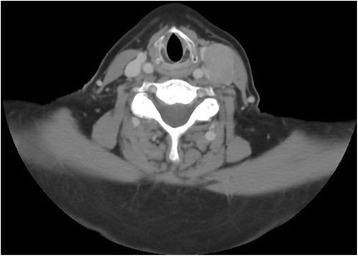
Computed tomography (transverse plane) revealing 2.8 × 1.7 cm mass in patient’s left lateral neck
